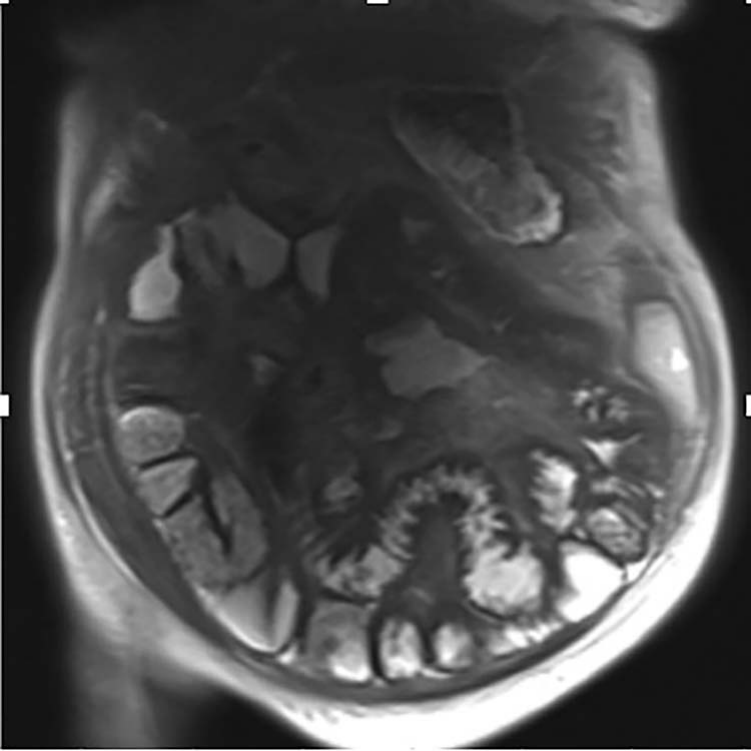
Coronal magnetic resonance image of abnormal thick-walled loops of jejunum and proximal ileum without focal stricture.
